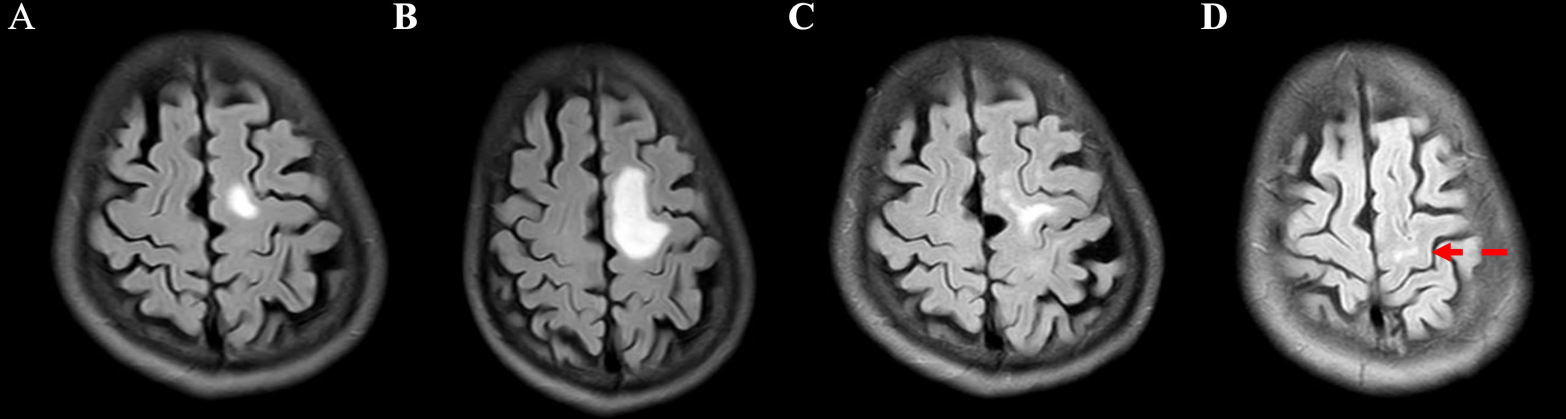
Brain MRI of the patient during the treatment process. (A) Initial imaging revealed a brain metastatic lesion about 1.2×1.0 cm in left frontal lobe. (B) After 7 months of crizotinib, the brain metastatic lesion size increased to 1.5×1.4 cm. (C) After radiotherapy and two cycles of camrelizumab, the brain metastatic lesion size reduced to 1.1×0.9 cm. (D) At 41 months from WBRT initiation (with subsequent camrelizumab), the brain metastatic lesion is almost undetectable (latest image).
